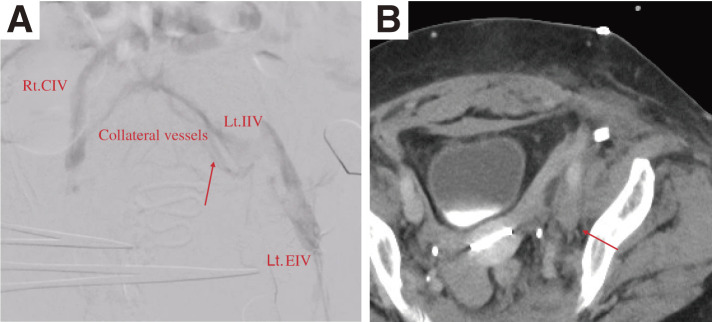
Postoperative outcomes. (A) Final intraoperative venography showing a patent venous flow from the external iliac vein to the contralateral iliac vein via collateral vessels and the internal iliac vein. (B) Postoperative contrast-enhanced CT in the delayed phase showing the patent external iliac vein and established collateral vessels leading to the anterior sacral surface (arrow), along with the resolution of the retroperitoneal hematoma without signs of further extravasation. CIV, common iliac vein; EIV, external iliac vein; IIV, internal iliac vein; Lt, left; Rt, right; CT, computed tomography
